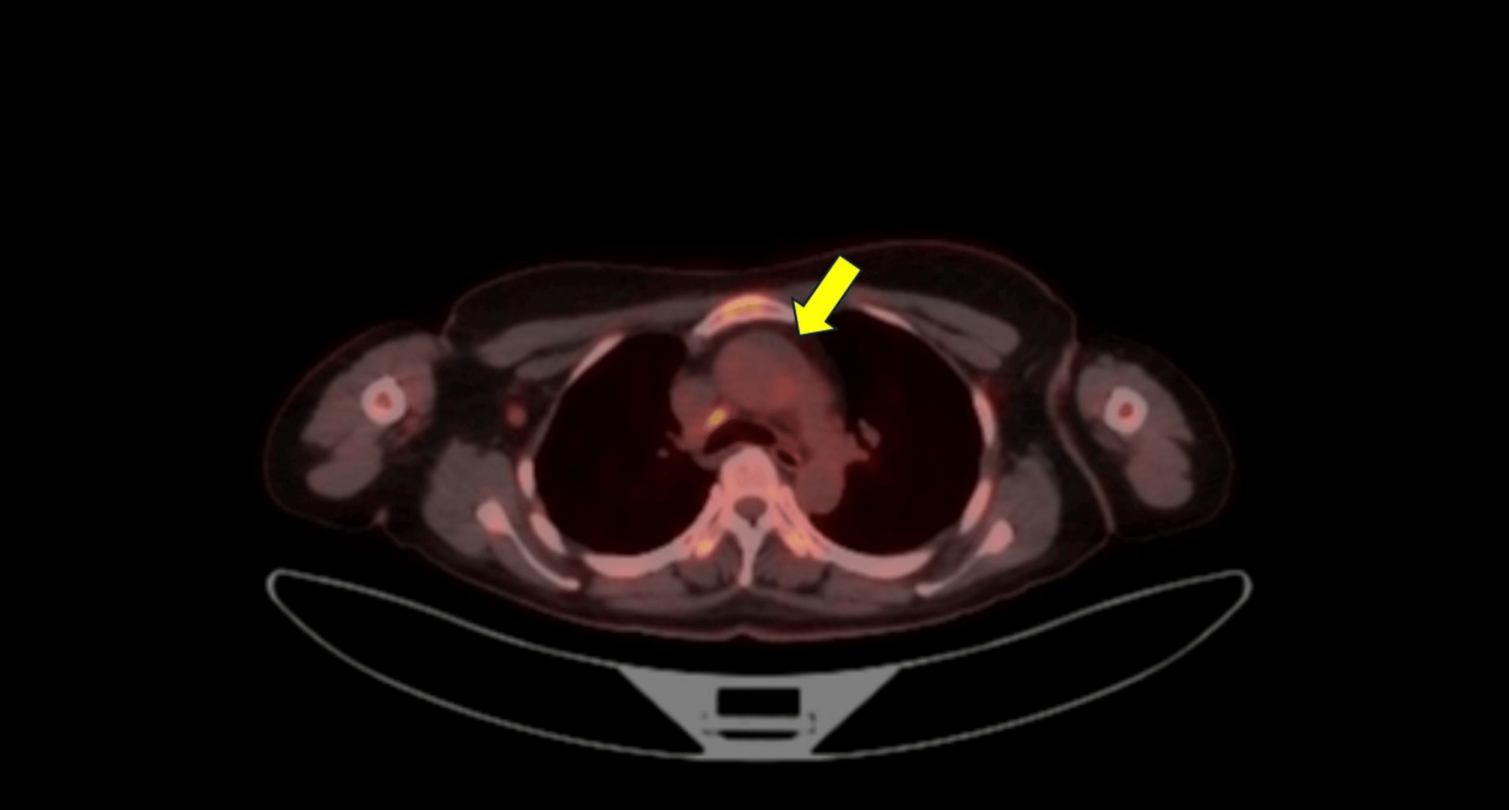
Axial view of FDG-PET/CT FDG-PET/CT showed no uptake in the area of the ascending aorta where wall thickening was observed on CT (arrow). FDG-PET/CT: fluorodeoxyglucose positron emission tomography/computed tomography
